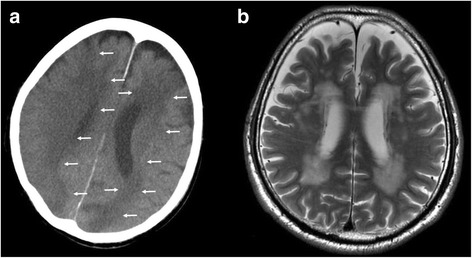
The postmortem CT and antemortem MRI (Case 2). a The postmortem CT image of the brain 2 h after death. LDAs were found widely, resulting in a diagnosis of cerebral infarction (*arrows*). (with permission [6]) b Antemortem MRI, T2-weighted image showing no cerebral infarction. The brain MRI was taken 1 year 5 months before death
